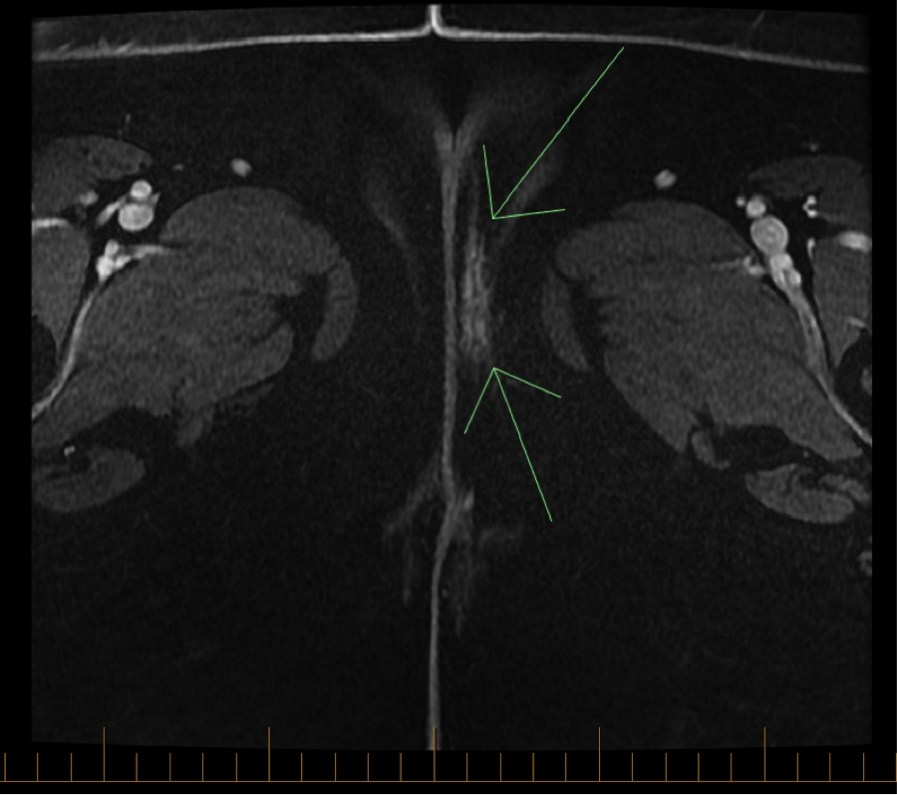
Axial MRI of the pelvis showing an abnormal enhancement in the left vulvar area measuring 3.7 cm in greatest dimension, representing tumor recurrence.
